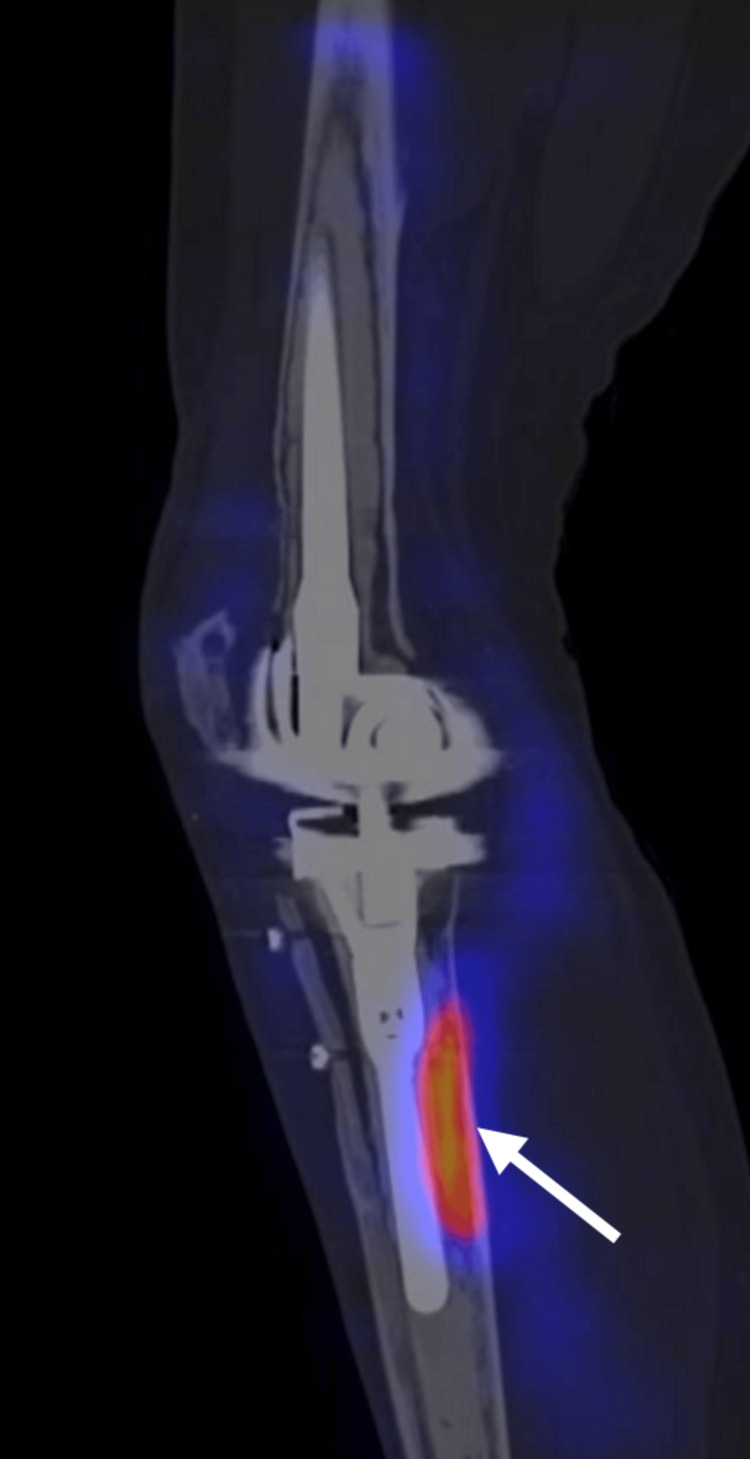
Antigranulocyte scintigraphy with SPECT of the right knee showing increased tracer uptake dorsolateral to the tibial stem 24 hours after injection SPECT: single-photon emission computed tomography
